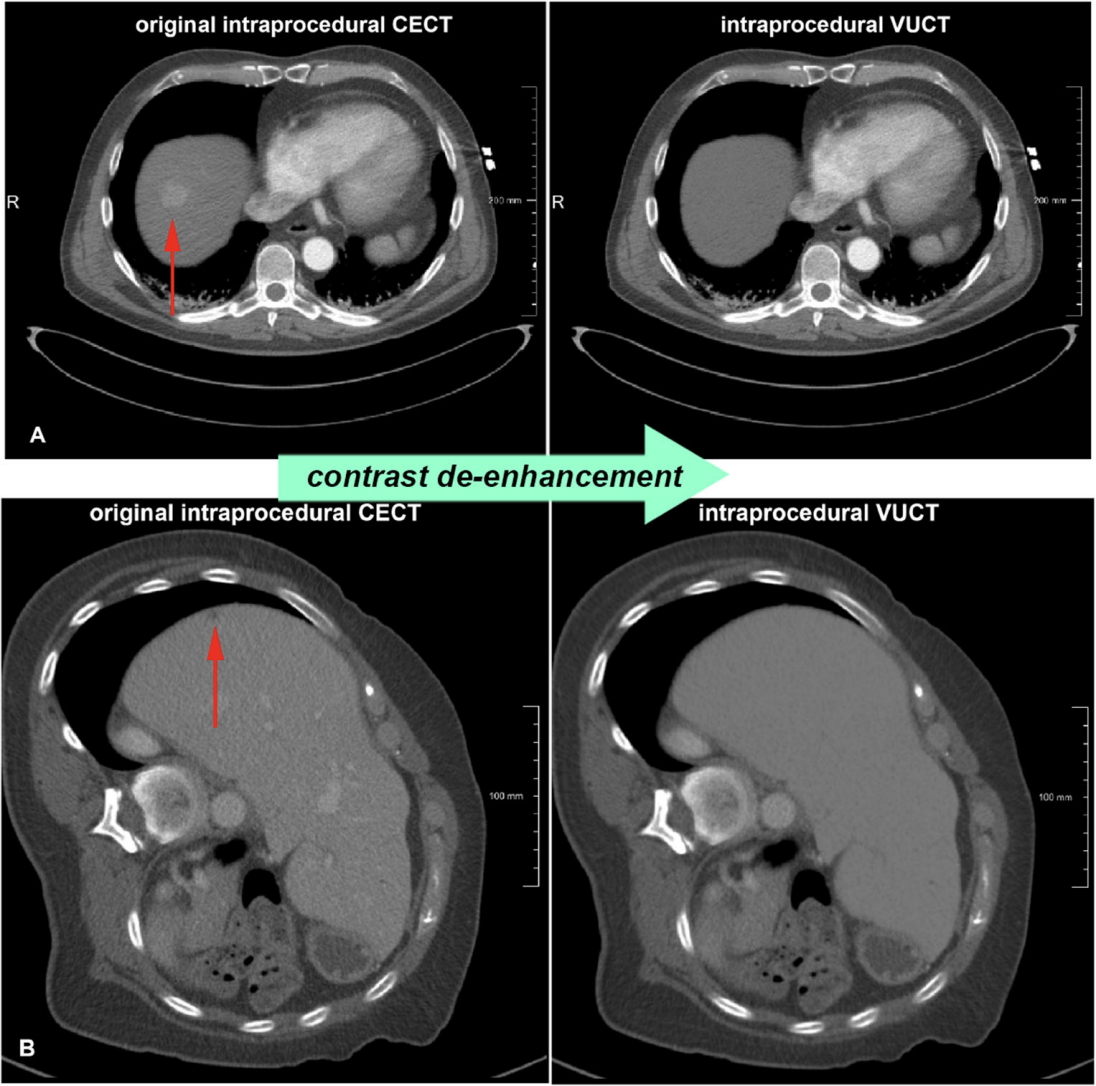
Examples of intraprocedural VUCTs based on contrast de-enhancement in the livers of intraprocedural CECTs. a An example of the virtual de-enhancement of the liver incorporating a hypervascular tumour (HCC). b An example of a hypovascular tumour (colorectal metastasis). Note that the contrast-enhanced vessels are also unenhanced. *VUCT* virtual unenhanced CT image, *CECT* contrast-enhanced CT image, *HCC* hepatocellular carcinoma
